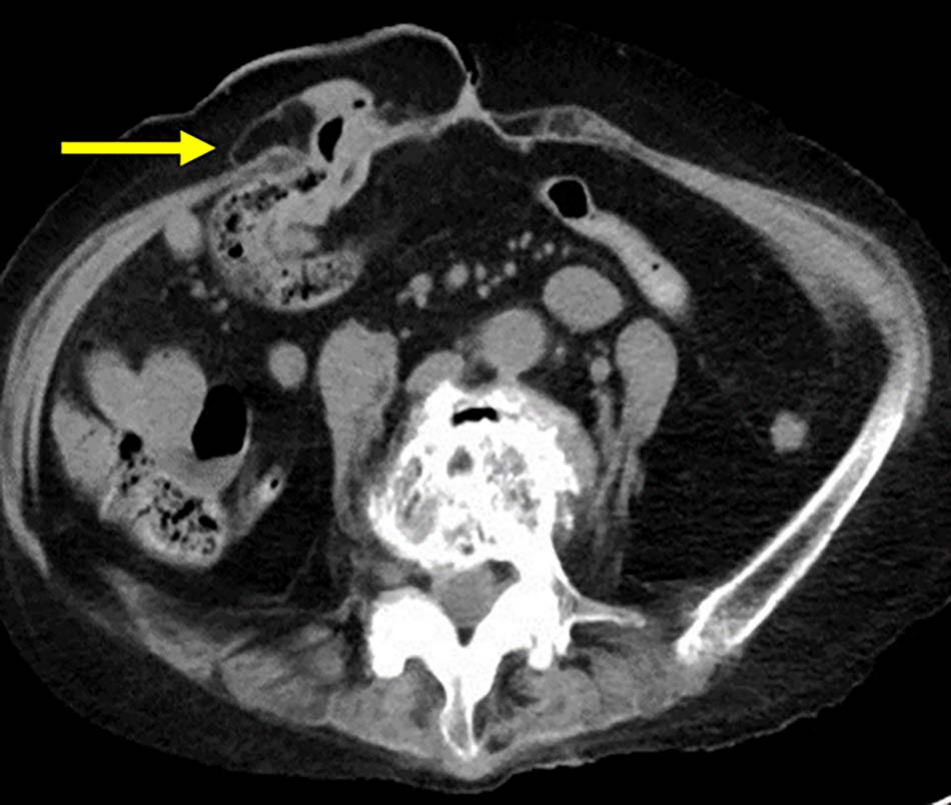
CT scan revealed no recurrence of the transverse colon on the oral side (arrow).
